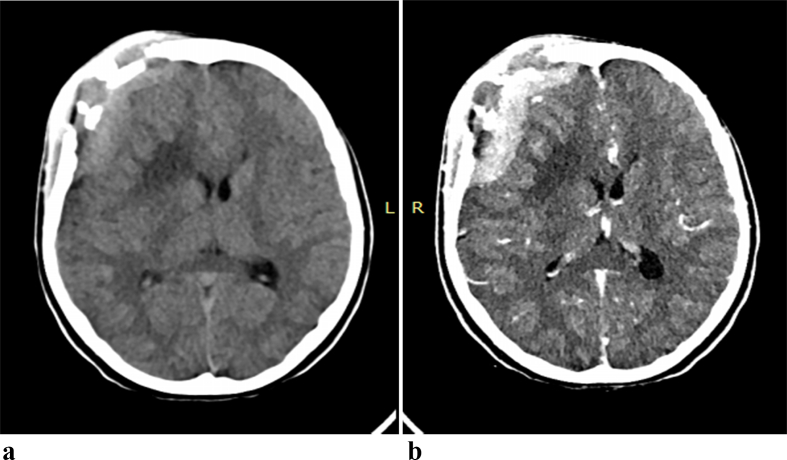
a. Axial non contrast computed tomography showing bony defect, with slightly hyperdense epidural and subgaleal lesion with mixed hypo dense areas plus adjacent brain parenchyma hypo density b. Axial contrast-enhanced computed tomography showing bony defect, with enhancing epidural and sub galeal lesion with non enhancing hypodense areas plus adjacent brain parenchyma hypo density.
